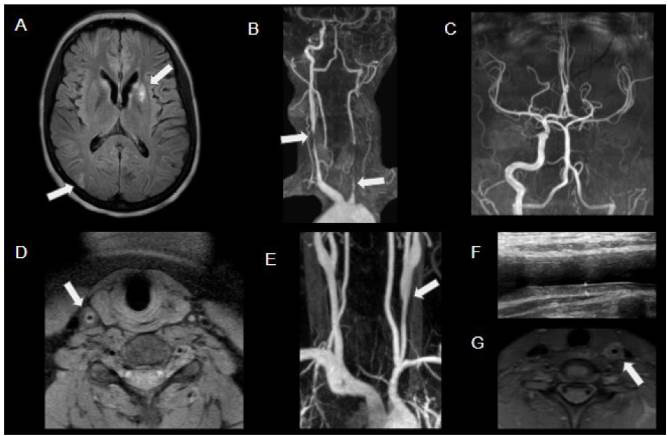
Takayasu’s arteritis in a patient with acute stroke in the left lenticulostriate region. Axial FLAIR (A) shows infarcted areas in the left nucleocapsular region and in the right posterior border zone (arrows). MRA imaging shows stenosis of the right common carotid artery, occlusion of the left common carotid artery (arrows), and also from both vertebral arteries, with distal filling by collaterals (B and C). Axial T1WI (D) shows circumferential thickening of the common right carotid artery (arrow). MRA (E) on another female teenager with Takayasu’s arteritis showing left common carotid stenosis (arrow). There was corresponding wall thickening on grayscale ultrasound (calipers in F) and marked wall thickening and enhancement on MR T1WI C+ FS (arrow in G).
